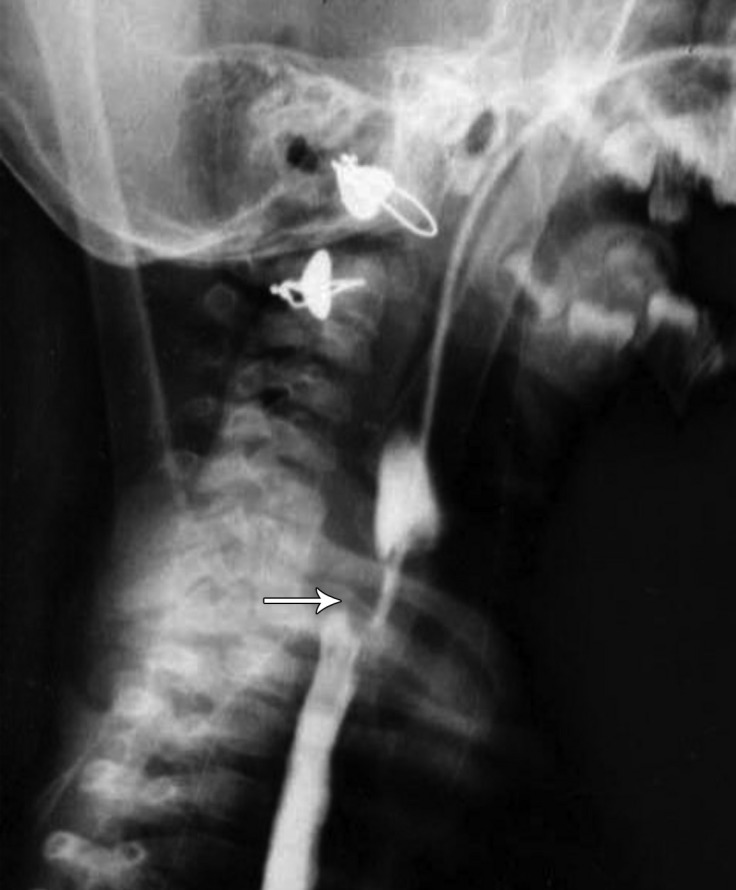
Patient’s barium meal, X-ray, showing pressure effects on the esophagus (arrow).
